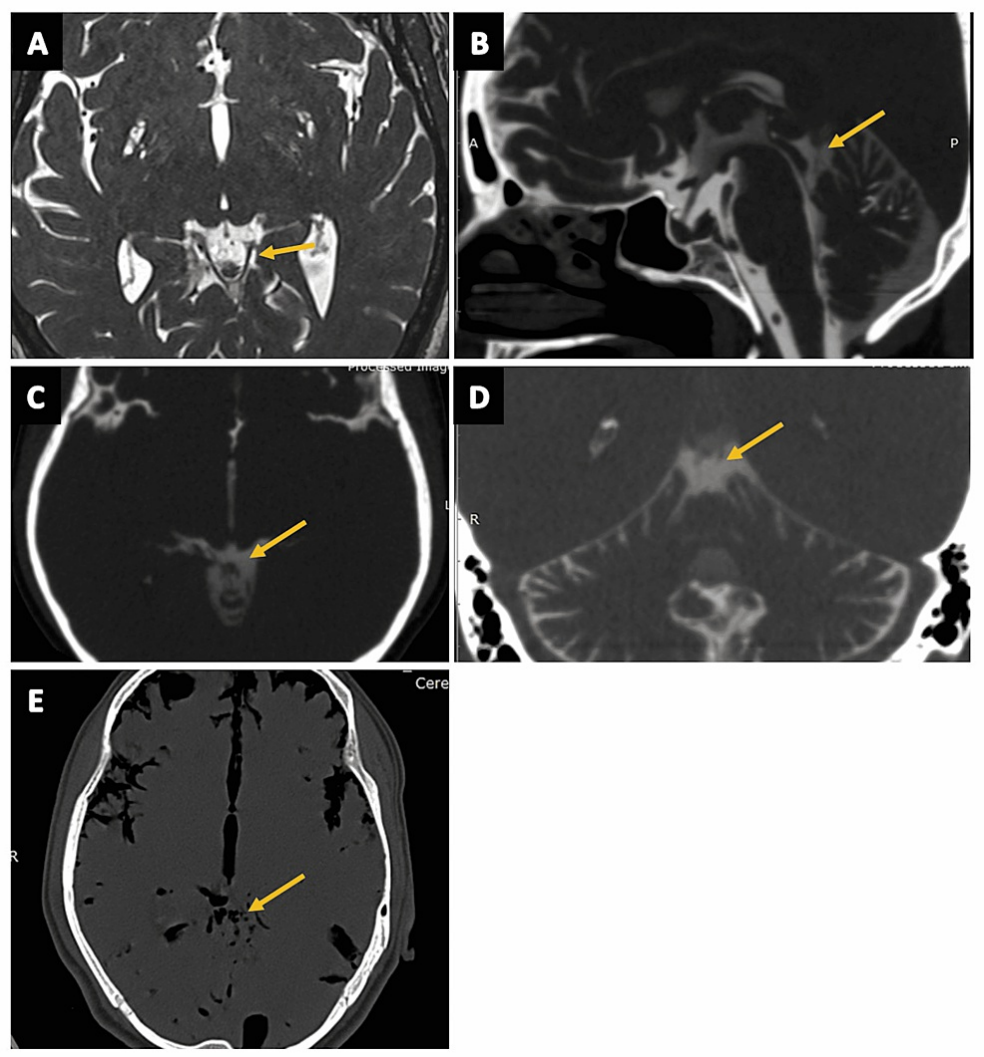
The superior cerebellar cistern. (A) The superior cerebellar cistern (arrow) as seen on the axial section of the three-dimensional (3D) constructive interference in steady state (CISS) MRI sequence. (B), (C), and (D) show the superior cerebellar cistern (arrows) as seen on the sagittal, axial, and coronal planes of CT cisternography, respectively, in a case of suspected CSF rhinorrhea. (E) The superior cerebellar cistern (arrow) as visualized in an axial plane of CT brain (negative contrast) in a case of pneumocephalus.
